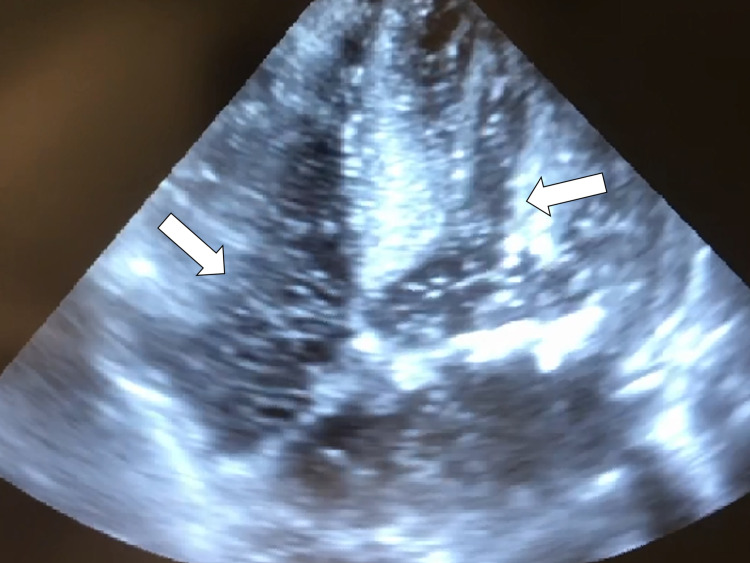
Echocardiography on the 17th day The echocardiography revealed microbubbles in bilateral cardiac cavities.
